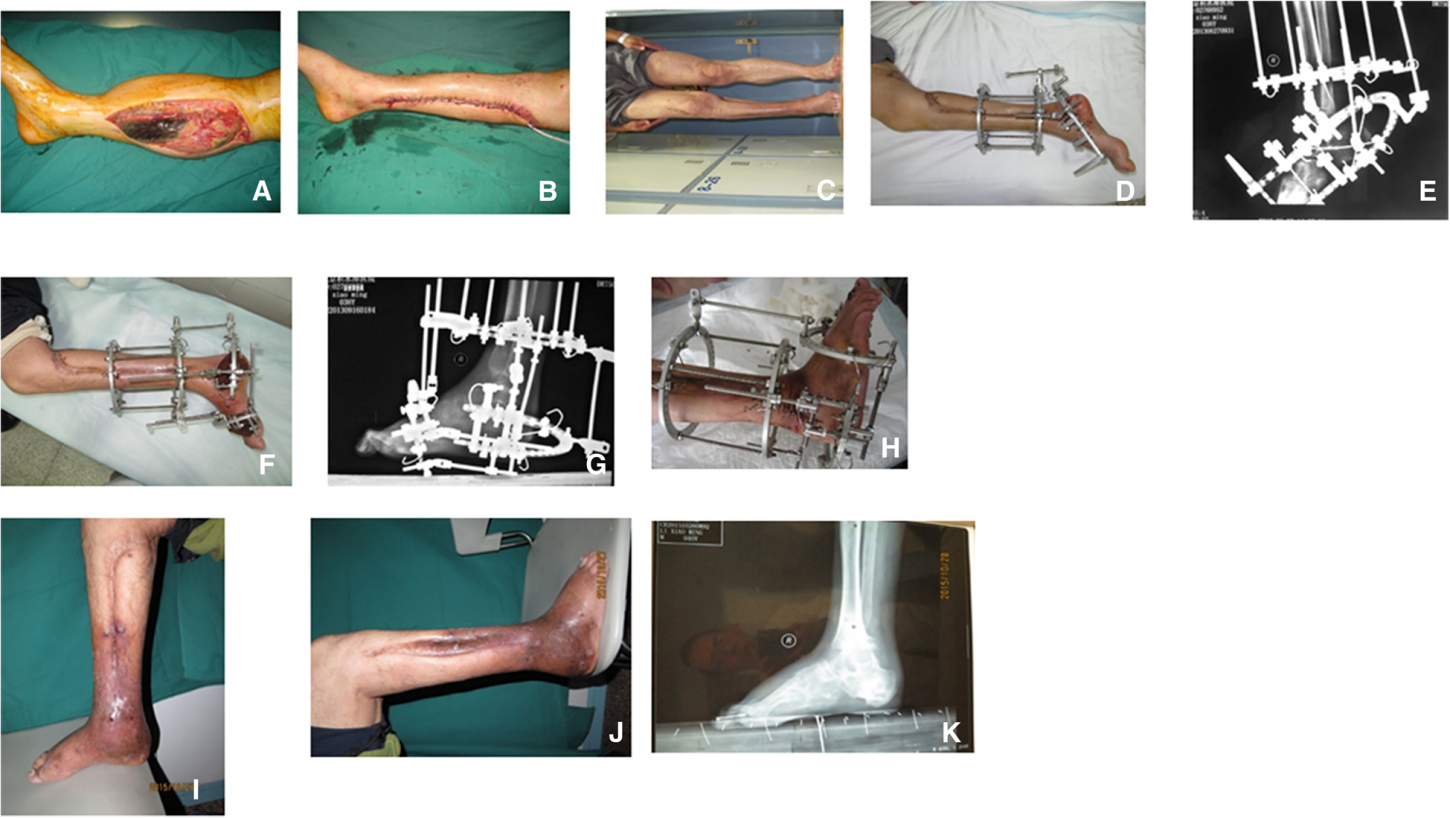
Representative images of one patient. a. The wound appearance after compartment fasciotomy of right lower limb. b. The wound was closed through skin suturation of right lower limb. c. The equinovarus deformity of right lower limb. d. The medial appearance after the application of Ilizarov fixator. e. X-ray image after the application of Ilizarov fixator. f. The ankle joint achieved 0。Dorsiflexion through the application of Ilizarov fixator. g. X-ray showed that ankle joint reached 0。Dorsiflexion. h. Image after the arthrodesis was performed. i. The equinovarus deformity was corrected completely (medial appearance). j. The equinovarus deformity was corrected completely (lateral appearance). k. X-ray showed that the equinovarus deformity was corrected completely
